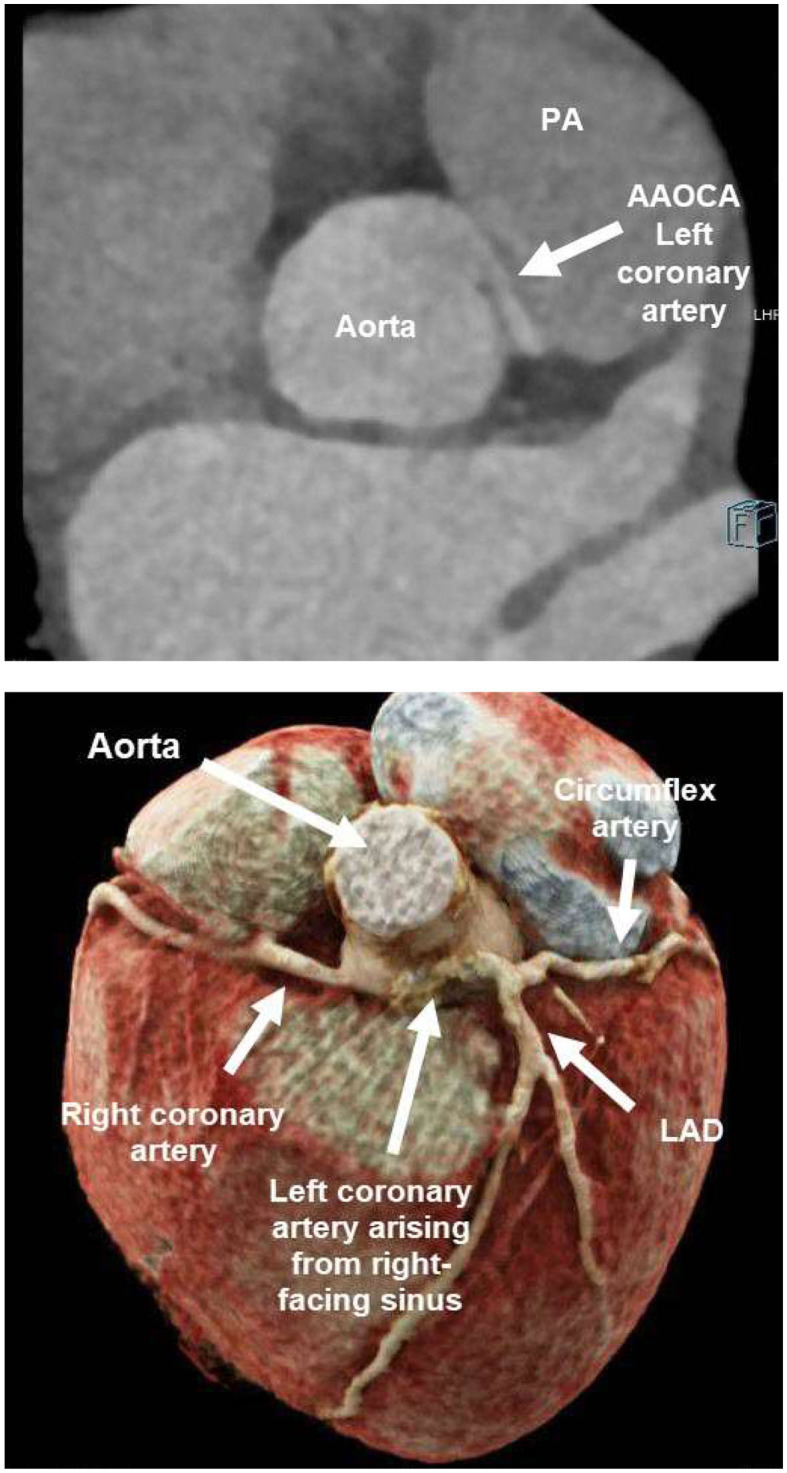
Ultra-low dose coronary computed tomography angiography (CCTA) of patient 1: anomalous aortic origin of the left coronary artery (LCA). LCA arising from the right-facing sinus with acute take-off angle, slit-like ostium and interarterial/intramural course between aorta and pulmonary artery (PA). Normal branching in left anterior descending (LAD) and circumflex artery.
